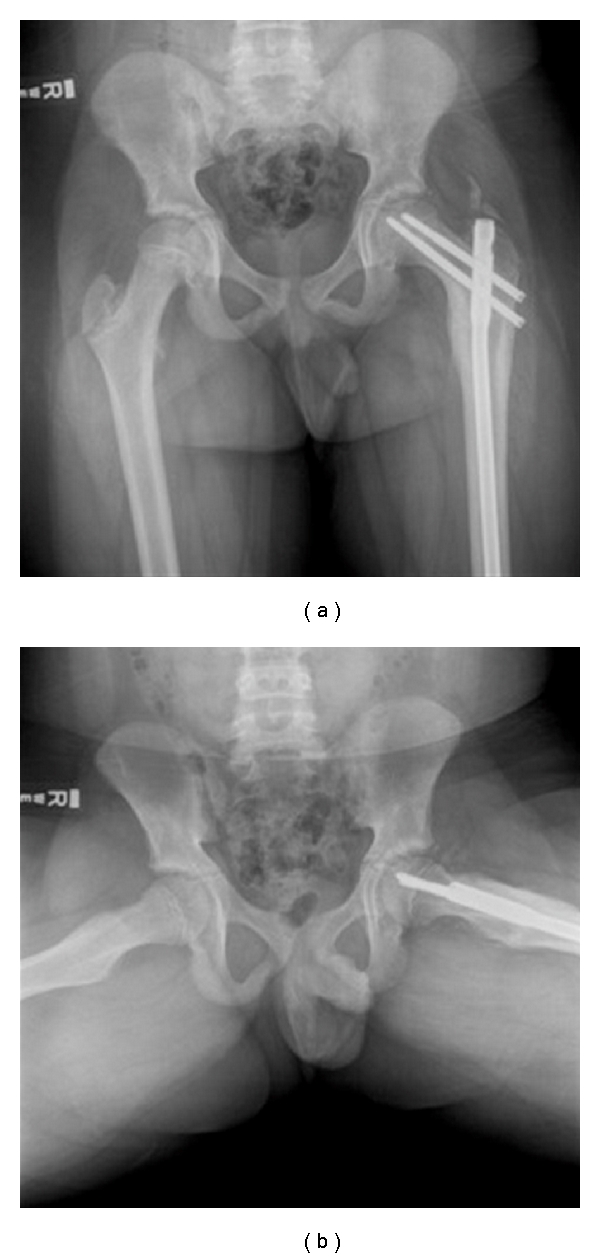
Radiographs 7 months after intramedullary fixation show a healed subtrochanteric fracture and no evidence of progression of the slip.
